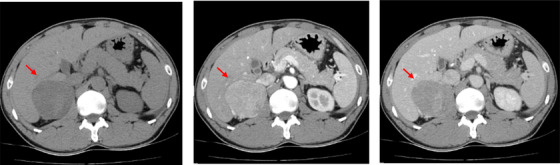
Adrenal CT scan. Left panel: routine scan; Middle panel: arterial phase; Right panel: venous and delayed phase
